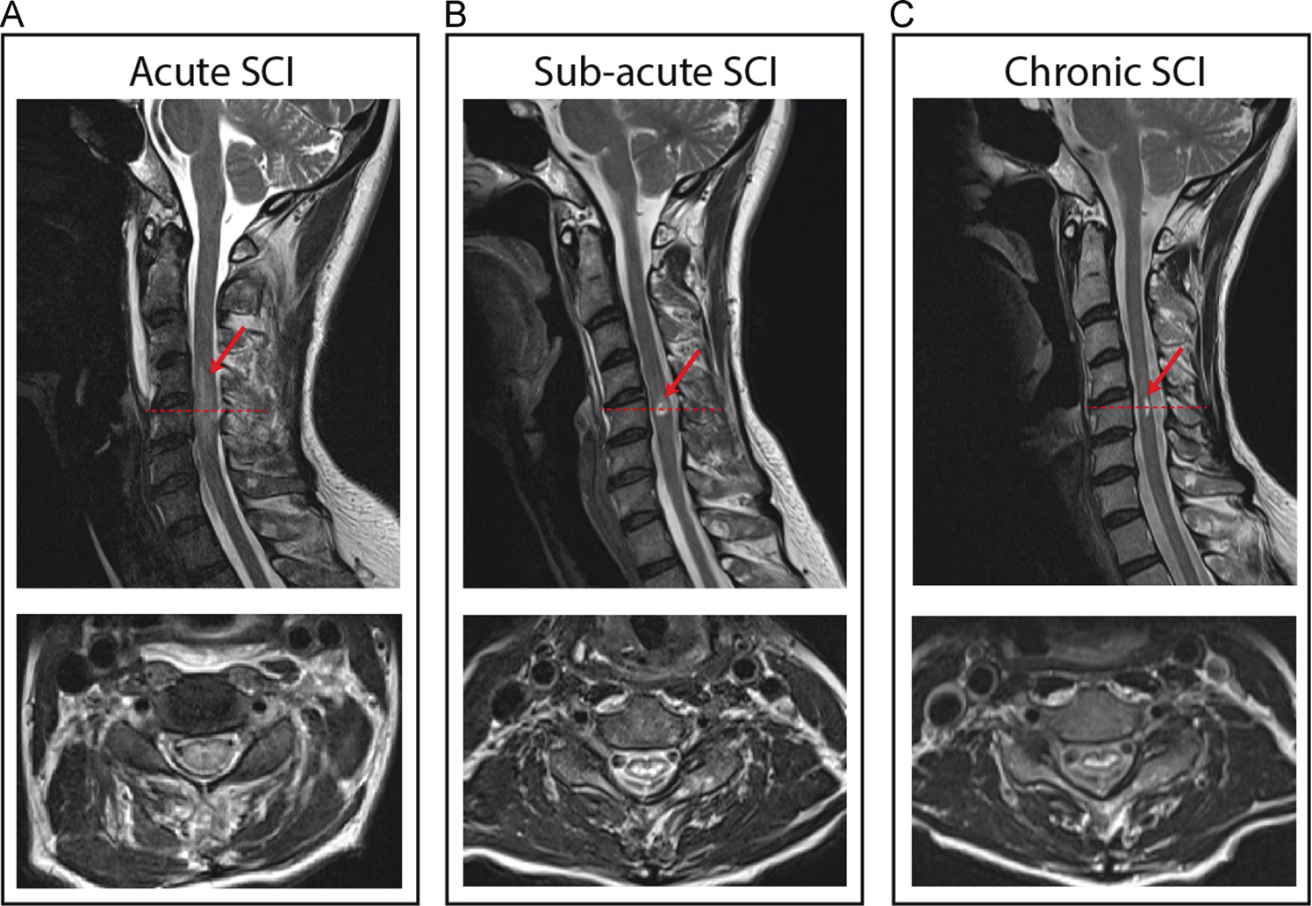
Overview of the lesion evolution with persisting midsagittal tissue bridges over time in a patient with traumatic cervical spinal cord injury. Sagittal and axial T2-weighted scans show the evolution of a cervical cord lesion from a 63-year-old  SCI patient (incomplete, female, AIS D) in a acute (1 day post-SCI), b subacute (1 month post-SCI), and c chronic phase (~24 months post-SCI)
